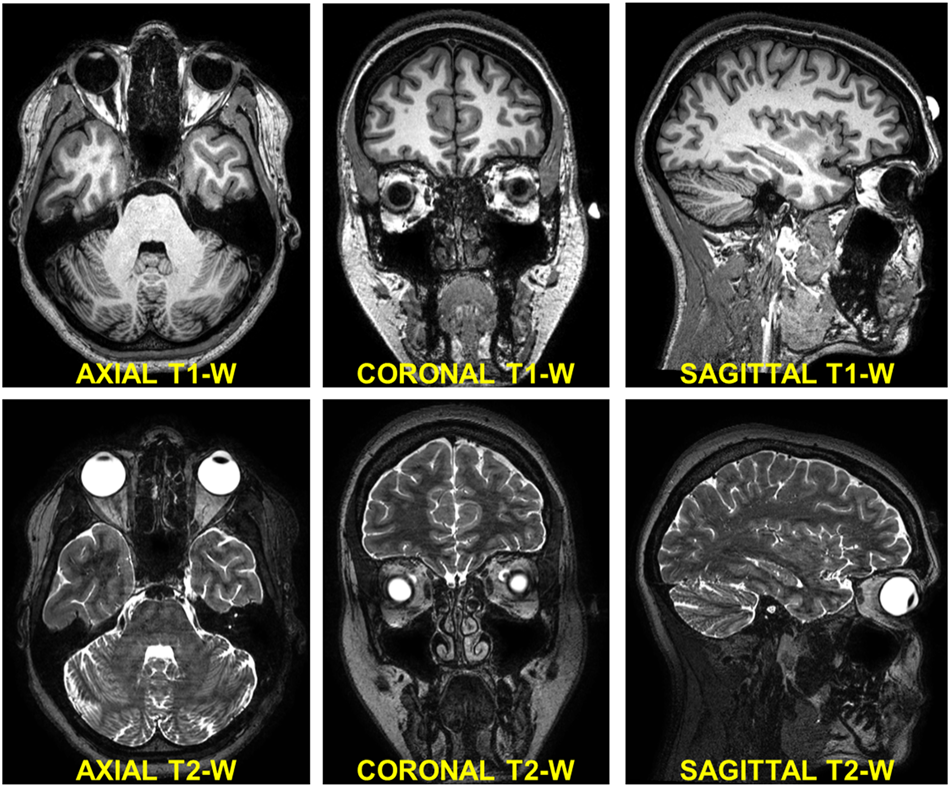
Structural MRI scans used for segmentation. Axial (left), coronal (middle), and sagittal (right) views of the T1- (top) and T2-weighted (bottom) structural MRIs. A specific T2-weighted MRI sequence with high nerve contrast optimized to enhance the structures of the ear and eye was also acquired (data not shown).
